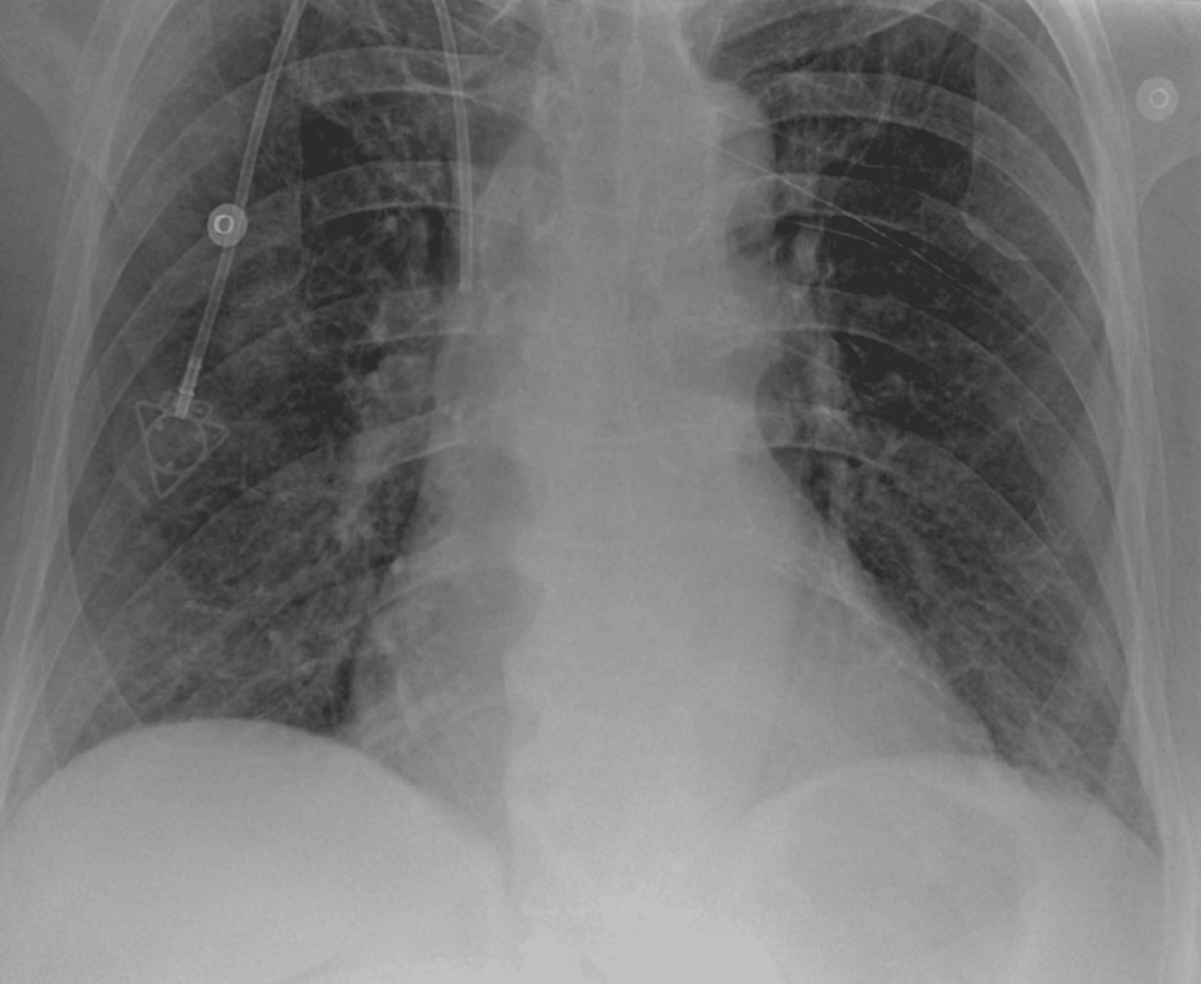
Chest X-ray showing the resolution of pleural effusion following the cessation of imatinib
